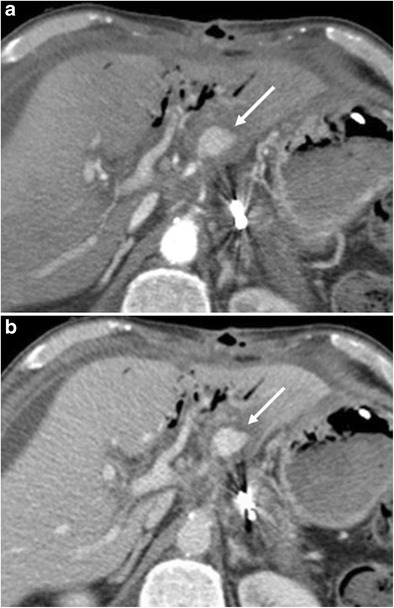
a, b) Axial CT images show an active extravasation in the arterial phase (white arrow in a) coming from the common hepatic artery after a Whipple procedure. Bleeding becomes more evident in the venous phase (white arrow in b)
